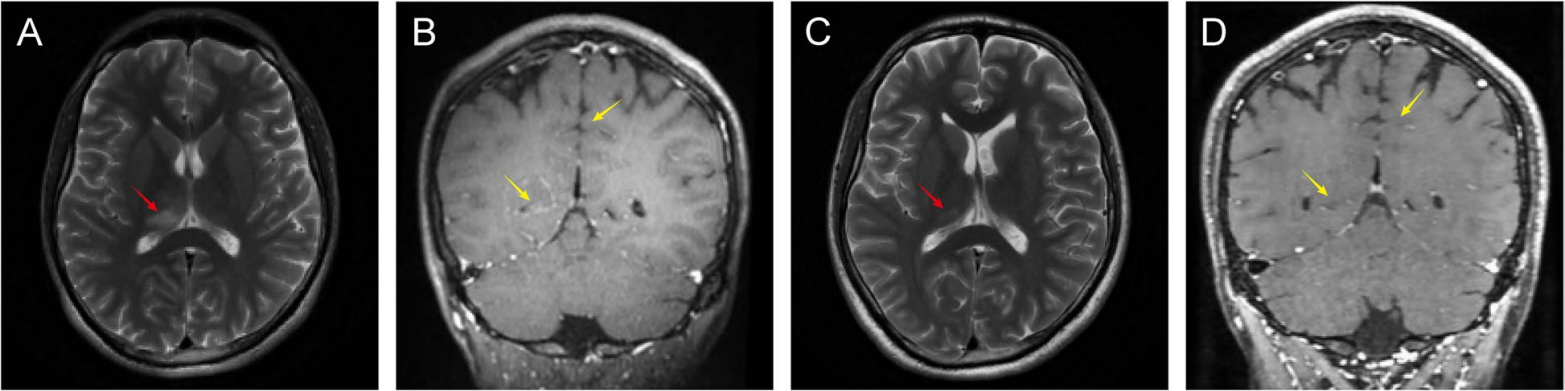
Brain MRI performance. A-B The MRI data of the patient 6 days after the symptom onset showed hyperintensity of the right thalamus on T2-weighted imaging (red arrows), and the pia mater is strengthened( yellow arrows). C-D Brain MRI scan performed 168 days after the initial symptom onset depicted a significant improvement in the imaging abnormality
